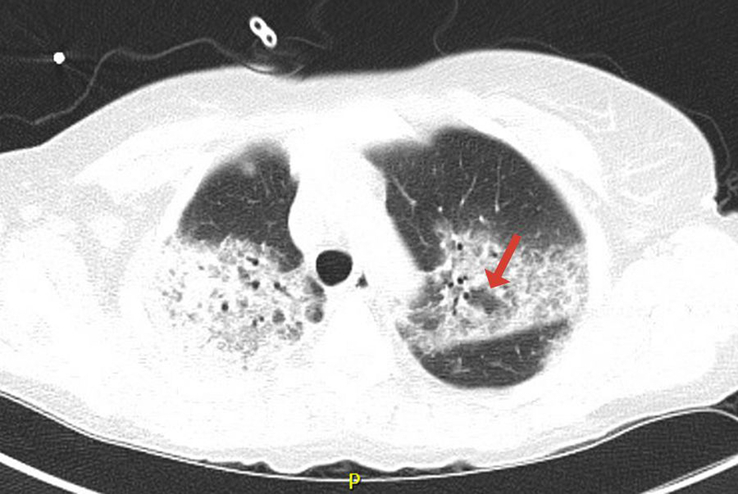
Computed tomography chest shows bilateral upper lobe consolidation, ground-glass opacities, interseptal thickening, and atoll sign (red arrow).
